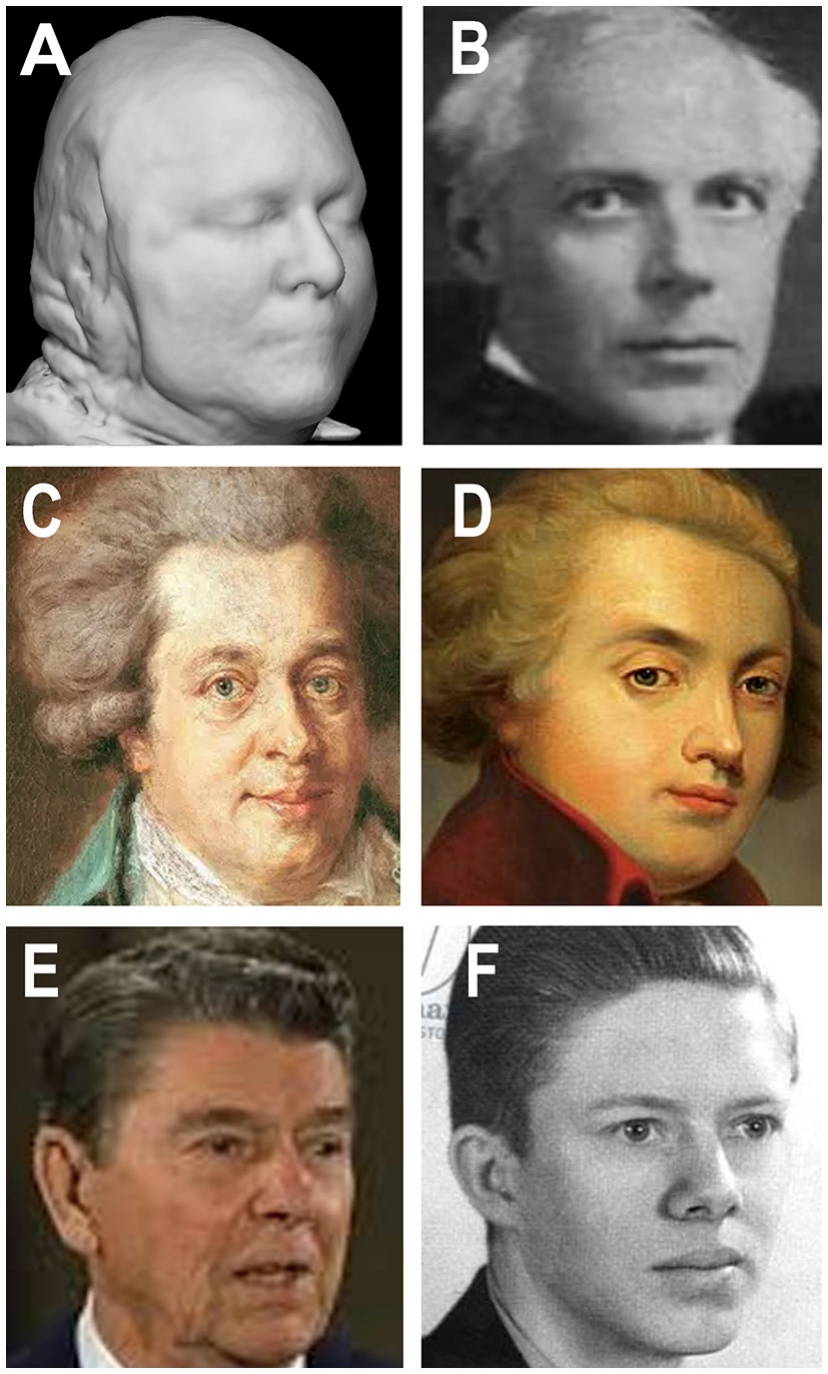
Brain magnetic resonance imaging (MRI) imaging data, composers, presidents, and the vagaries of facial identification. (A) Reconstructed image from a modified de-faced MRI image (Schwarz et al., 2021). (B) Hungarian composer Béla Bartók (1881–1945) showing similarities to panel (A). (C, D) Viennese composer Wolfgang Amadeus Mozart (1756–1791) in two depictions that are poorly similar to each other even though they are of the same person. (E, F) American Presidents: Ronald Reagan (1911–2004) and James (Jimmy) Carter (1924–) showing greater or lesser similarity to (A), but millions of other images show equal similarities. The similarities and disparities argue that properly de-faced MRI imaging data, even if partly reconstructed (A), are insufficent to identify any specific individual. (A) Reproduced with permission from Christopher Schwarz (Mayo Clinic, Rochester, USA). (B) (https://en.wikipedia.org/wiki/B%C3%A9la_Bart%C3%B3k) and (C) (https://en.wikipedia.org/wiki/Horn_Concerto_No._1_%28Mozart%29) were granted permission to reproduce under the Wikipedia Creative Commons Attribution-ShareAlike 3.0 Unported License. (D) (https://cdn.fansshare.com/photo/wolfgangamadeusmozart/wolfgang-amadeus-mozart-full-image-1140486490.jpg) is reproduced with permission under the FansShare Corporation Copyright and Intellectual Property Policy. (E) (https://www.shutterstock.com/editorial/image-editorial/ronald-reagan-396347ci) is reproduced for academic use only with permission from Shutterstock, reference Jeisson 27/12/2022. (F) (https://cdn.shopify.com/s/files/1/0720/2785/products/4637.jpeg?v=1571265455) is reproduced under the terms of https://burst.shopify.com/ that grants free reproduction for academic purposes.
